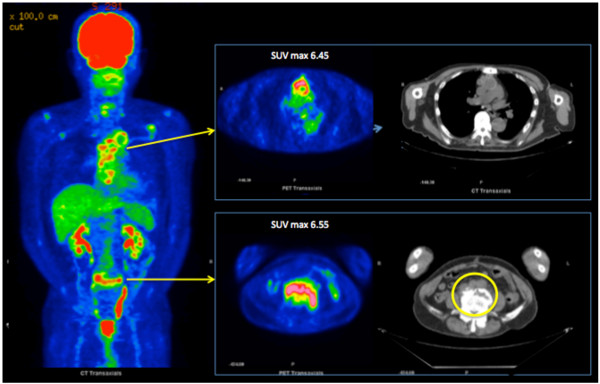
^18^ F-FDG-PET/CT in Case 1.*Circle*; Abnormal uptake of ^18^ F-FDG in the upper part of the 5^th^ lumbar vertebra (SUVmax 6.55); osteolysis was evident at the same site on CT. *; Although substantial ^18^ F-FDG accumulation was seen around the ascending and arch aortic graft, it was judged as physiological.
